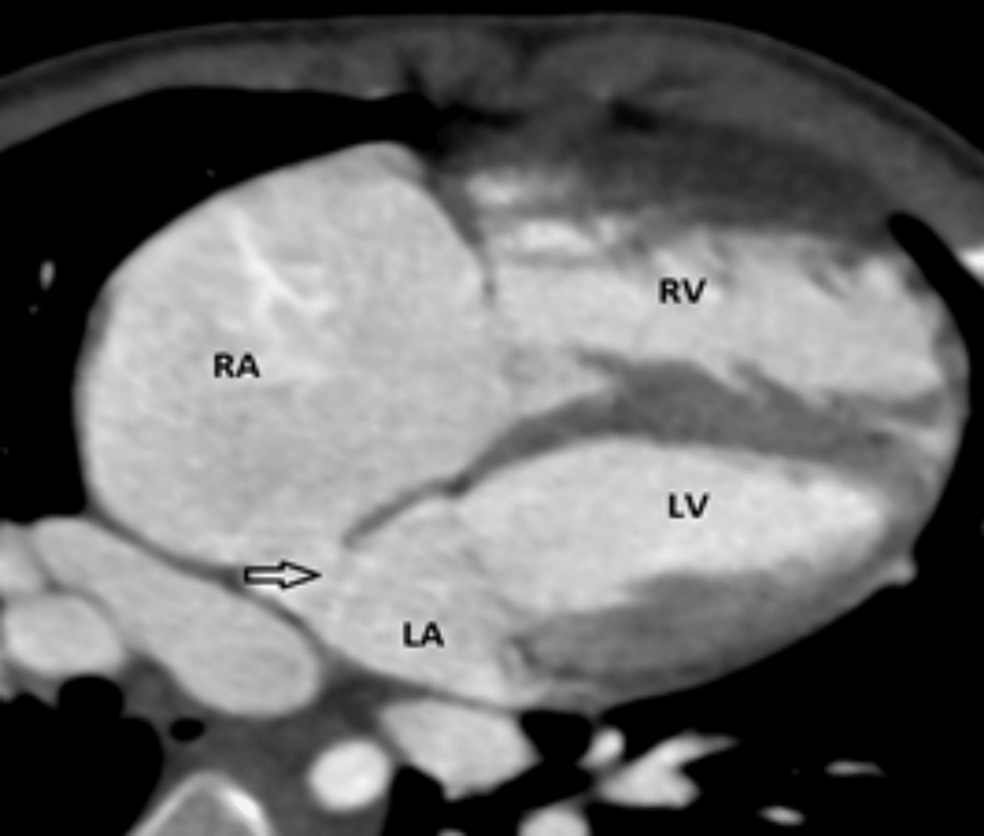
Cardiac CT axial section showing dilated RA and RV; there is also an atrial septal defect (black arrow) resulting in a right-to-left shunt CT: computed tomography, RA: right atrium, RV: right ventricle, LA: left atrium, LV: left ventricle
